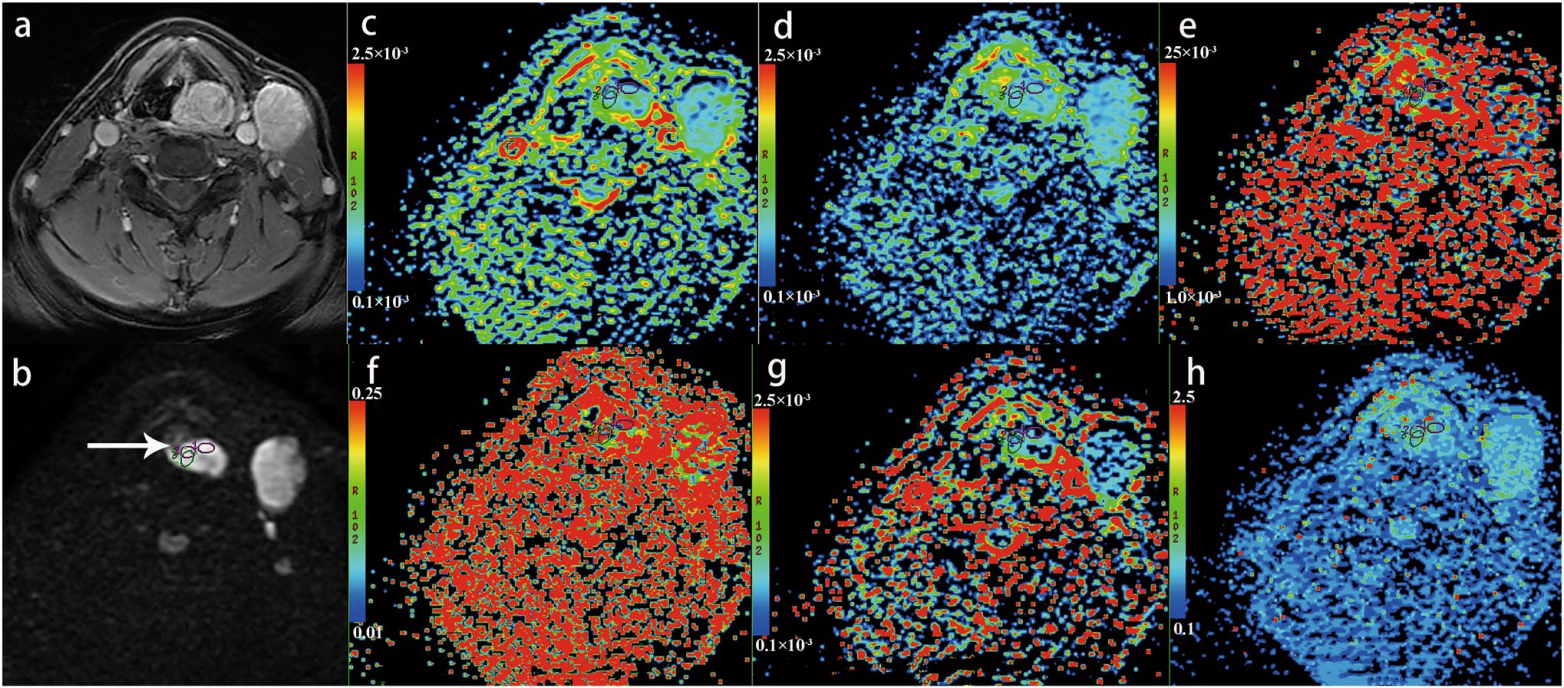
A 57-year-old man with hypopharyngeal squamous cell carcinoma is presented. Over 14 months of follow-up, the patient developed lymph node metastasis. Axial contrast-enhanced T1-weighted image (a) shows an obviously enhanced primary tumor at left piriform fossa and nodal mass at levels II and III of neck. b IVIM image (*b* = 800 s/mm^2^ level) shows the mass was hyperintense (white arrow). The three circles presented in the mass are the regions of interest (ROIs) sketched artificially in the typical area of the largest level of the mass, and are applied to each functional map. Apparent diffusion coefficient map (ADC) c, ADCmean = 0.58 × 10^−3^mm^2^/s, ADCmax = 0.831 × 10^−3^mm^2^/s, ADCmin = 0.12 × 10^−3^mm^2^/s, ADCrange = 1.218 × 10^−3^mm^2^/s; true diffusion coefficient (D = 0.673 × 10^−3^mm^2^/s) d, pseudo diffusion coefficient (D* = 67.5 × 10^−3^mm^2^/s) e, perfusion fraction (*f* = 26.6%) f, distributed diffusion coefficient (DDC = 0.513 × 10^−3^mm^2^/s) g and diffusion heterogeneity index (*α* = 0.729) h maps are shown.
